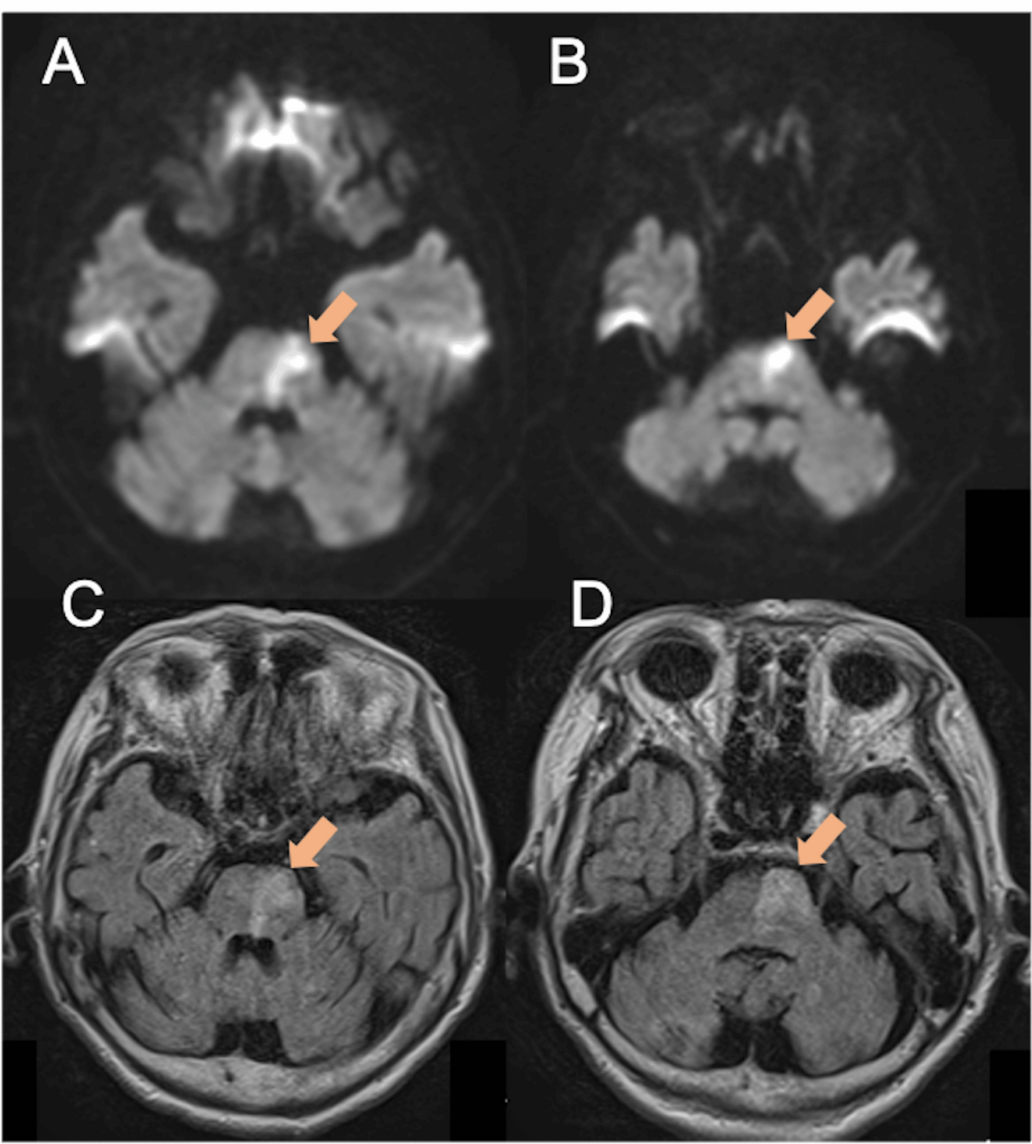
Magnetic resonance imaging (MRI) at symptom onset, including diffusion-weighted imaging (DWI: A,B) and fluid-attenuated inversion recovery (FLAIR: C,D) sequences The infarction was located in the left paramedian artery territory and involved the corticospinal tract, pontocerebellar fibers, medial lemniscus, reticular formation, superior cerebellar peduncle, and vestibular nuclei.
